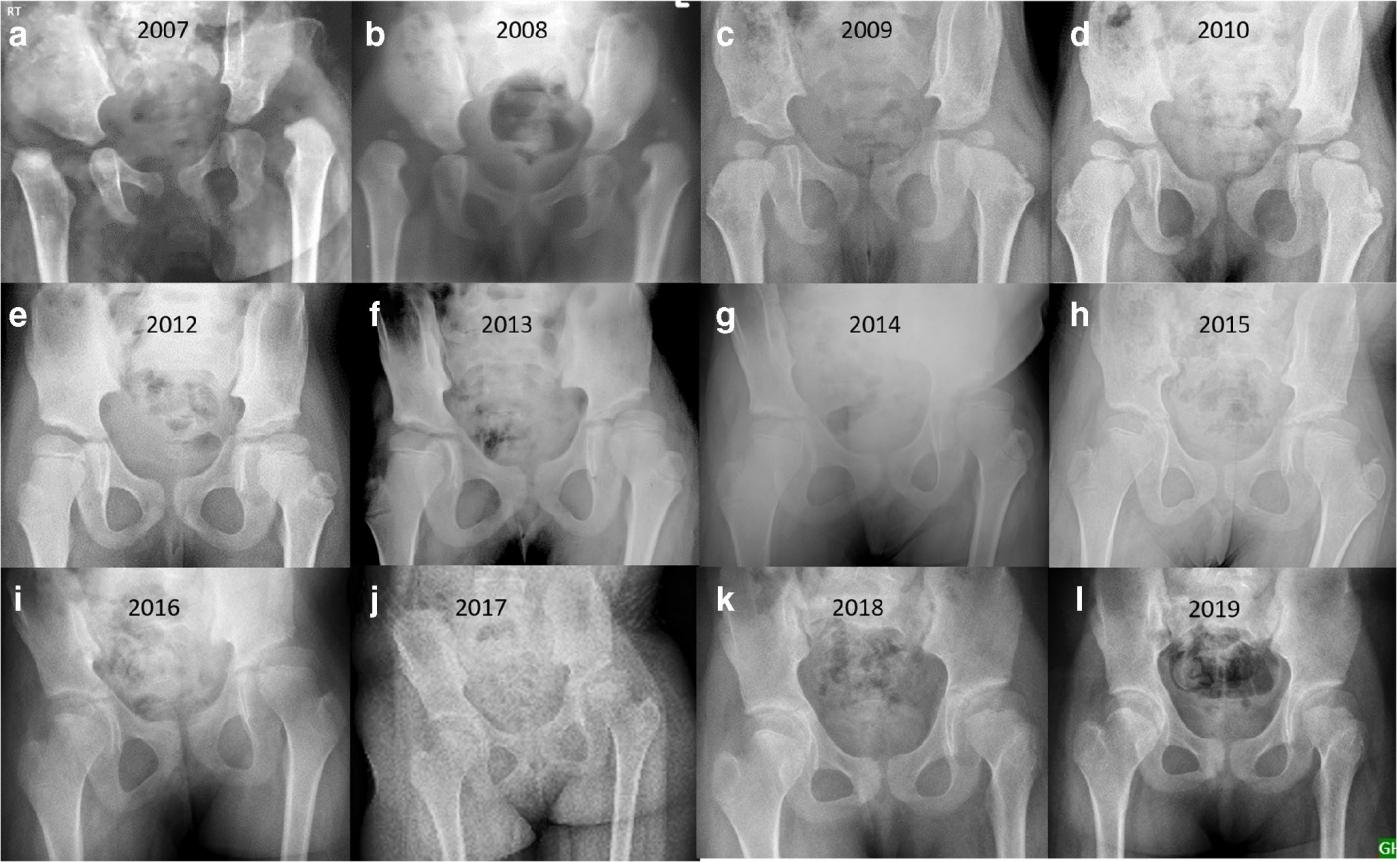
Serial radiographs of a child without ligamentous laxity showed good outcomes 12 years after surgery (a–l). Lateral growth arrest of the proximal femur physis was present with the excellent remodeling of the acetabulum
